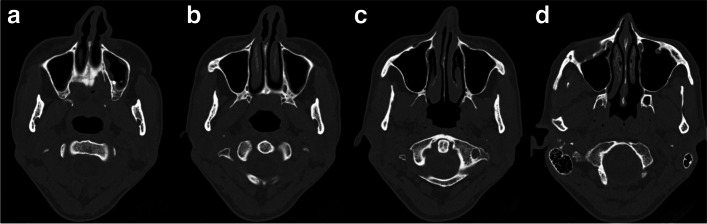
CT of the external nose and nasal cavity of the patient A, depicting a asymmetric tip, narrow right external valve with weak left external valve, thickened soft tissues of septum, and inwards rotated lateral crus of lower lateral cartilage bilaterally; b hypertrophic lower turbinates, septal deviation, and adhesion between septum and right lower turbinate; c collapsed internal nasal valves and subtle septal spur; and d inwards rotated nasal bone following too high osteotomy, wide high septum, and concha bullosa bilaterally
